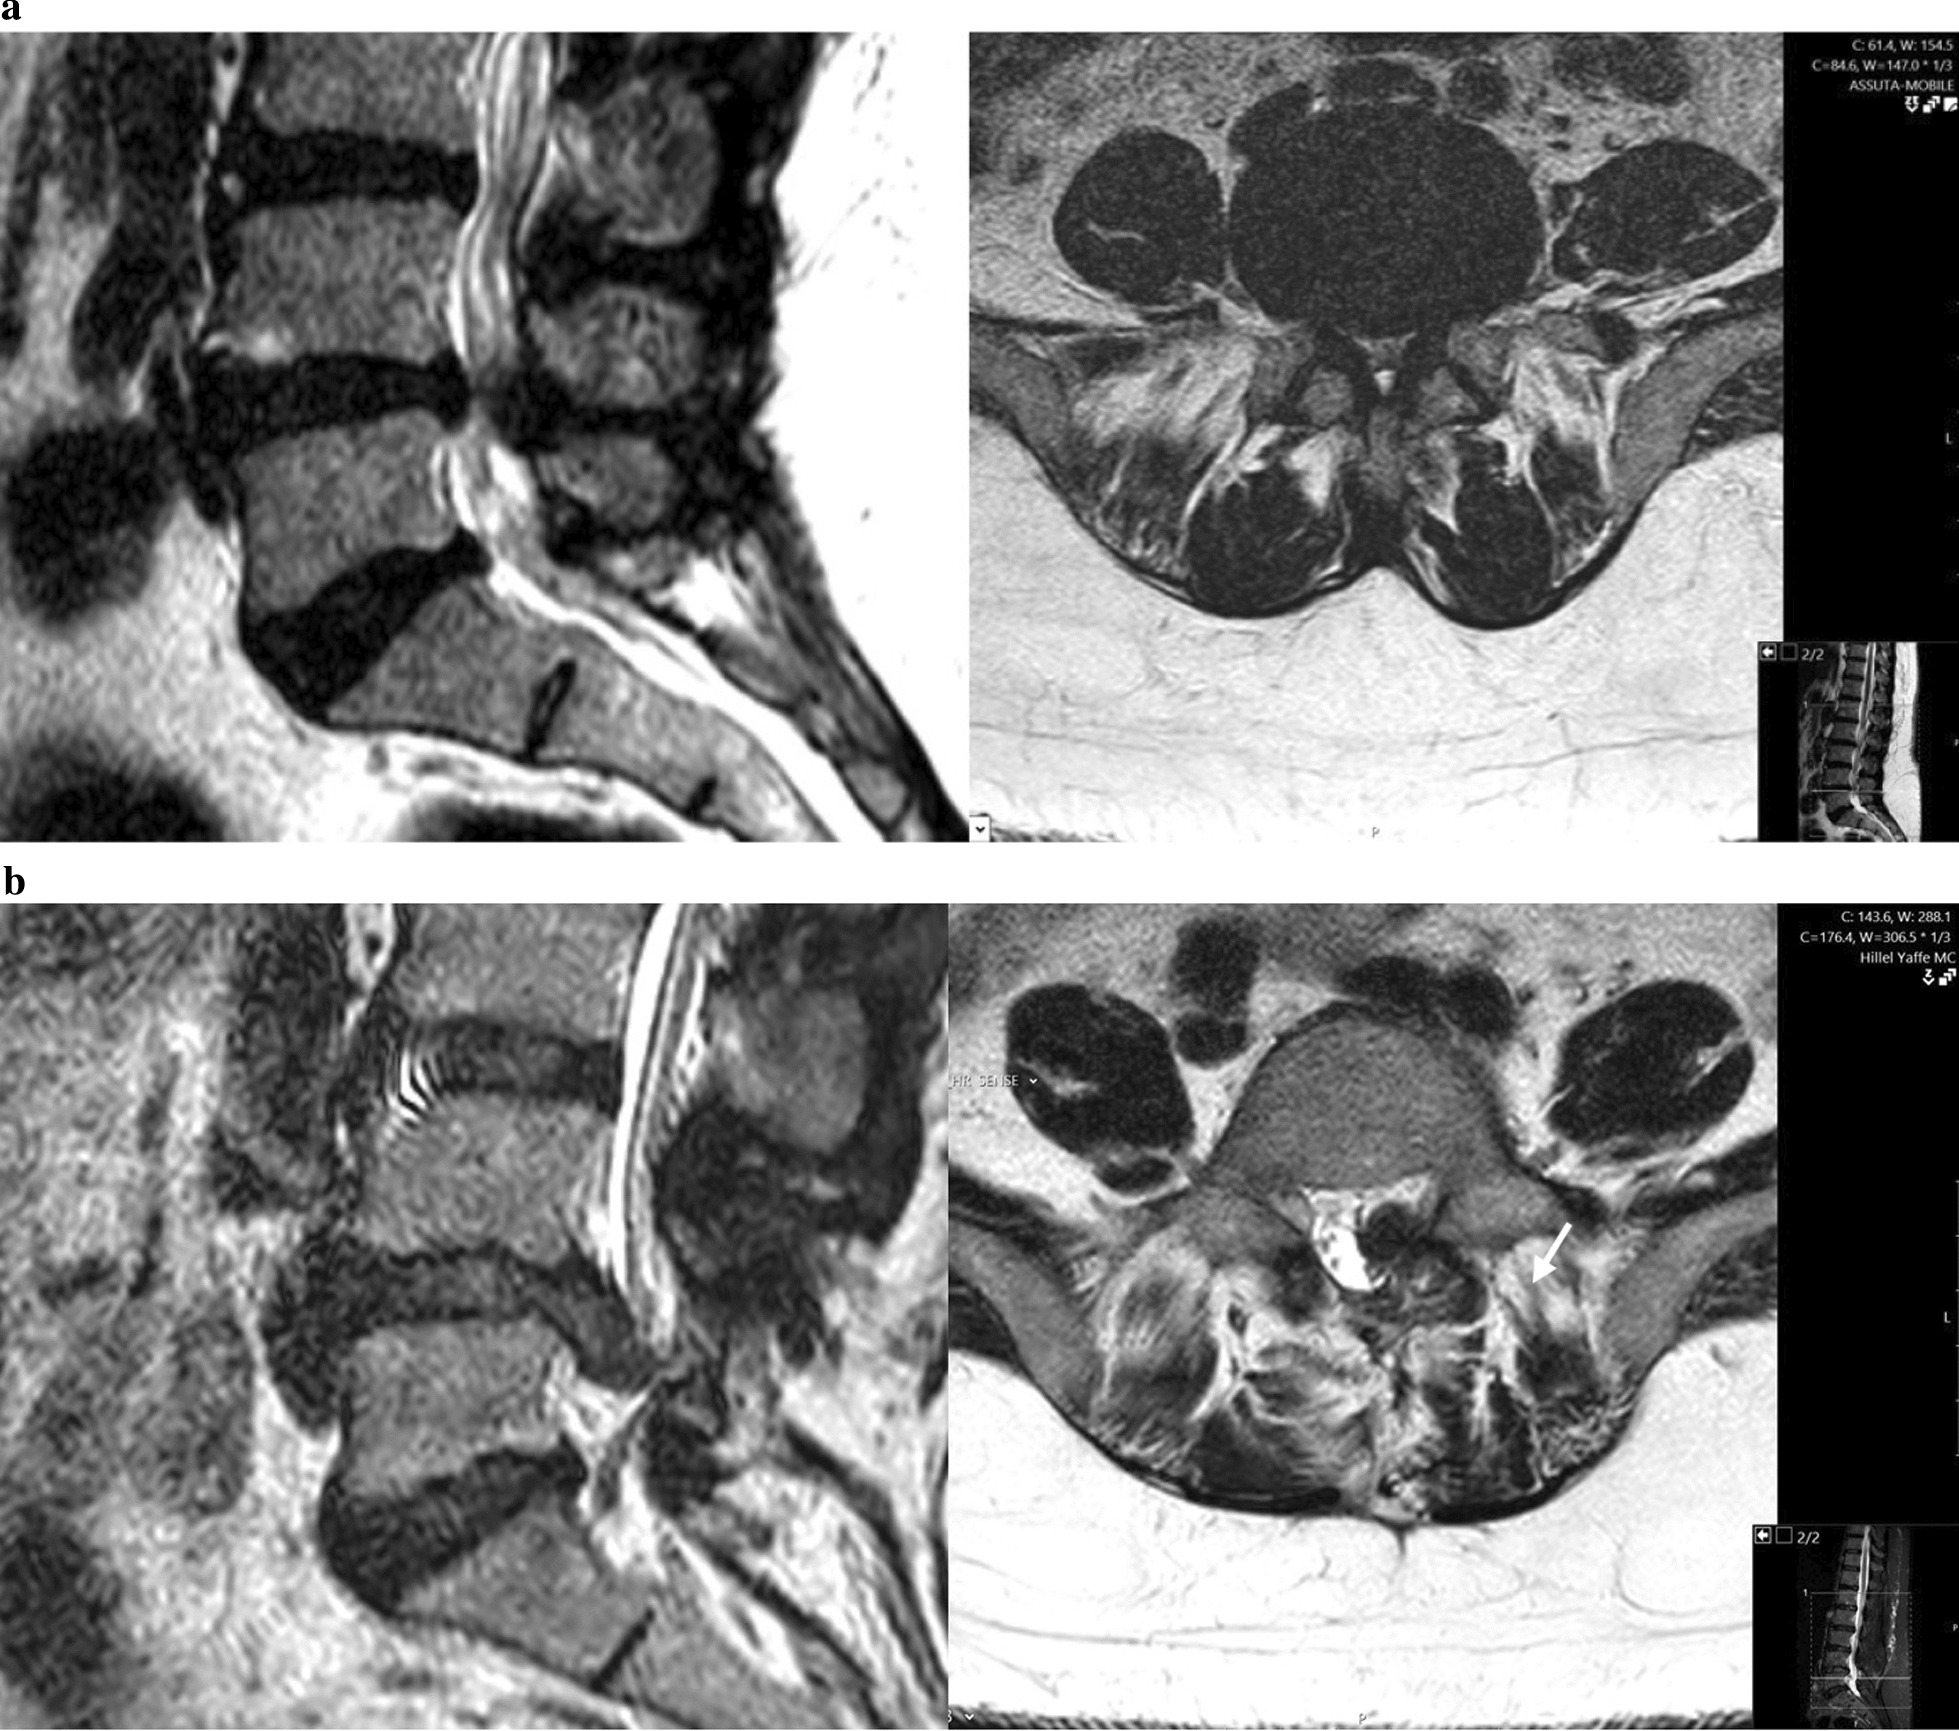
a Preoperative lumbar MRI images of a 62-year-old male with chronic bilateral L5 radicular pain and neurological claudication due to severe L4–5 spinal stenosis. b Postoperative MRI images of the same patient following mini-open decompression (involving bilateral partial laminectomy) demonstrating acute paracentral disc herniation
